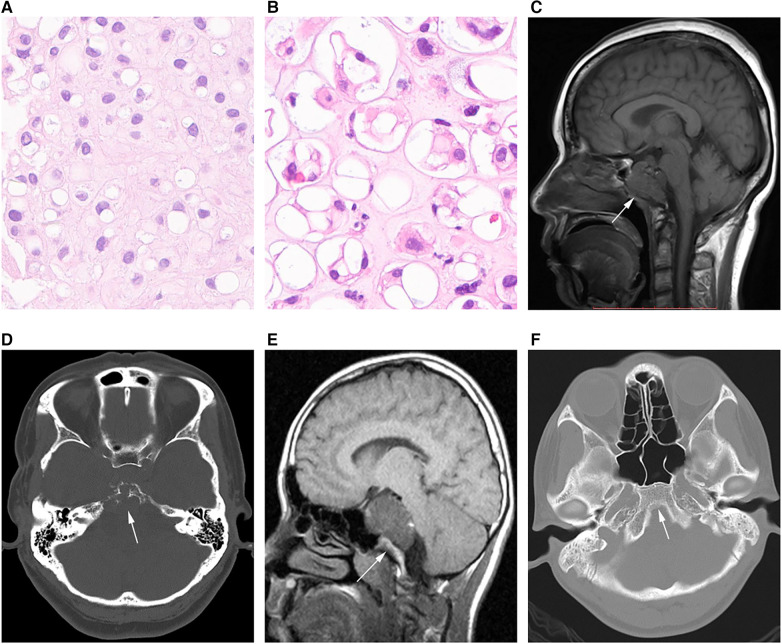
Different chordoma types. (A) Pathological image of classical chordoma showing many large cells with clear to eosinophilic cytoplasm; physalides are common in tumor cells. (B) Image of chondroid chordoma showing structures similar to the cartilage matrix between tumor cells. (C,D) MRI and CT scans in an SBC case showing that the tumor grows with the invasion of the whole clival bone (arrow). (E,F) CT image in another SBC case, with no bone invasion (arrow).
